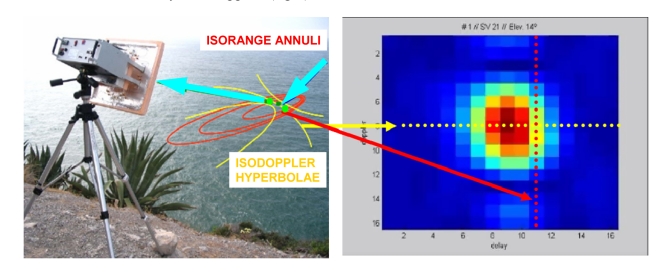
(left) GNSS-R geometry of observation from Garraf coast (South of Barcelona). Red: isorange annuli (same delay), Yellow: isoDoppler hyperbolae (same Doppler), Green dots with same delay and Doppler. (right) measured DDM.
